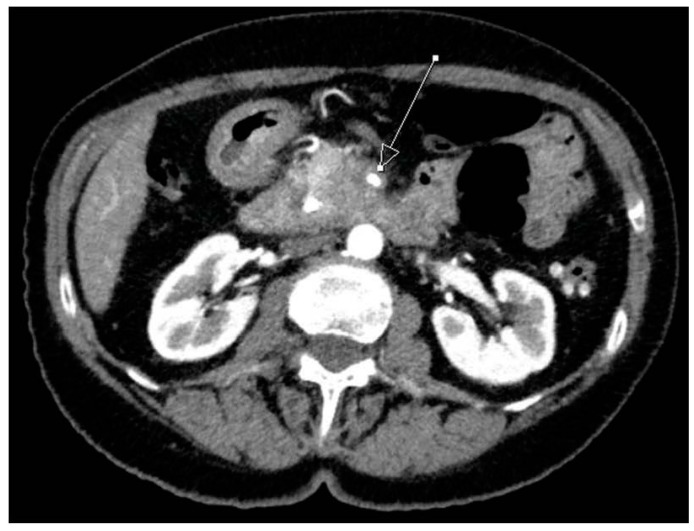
Computed tomography image of a tumor in the pancreatic head which surrounds the superior mesenteric artery (arrow: “unresectable”).
